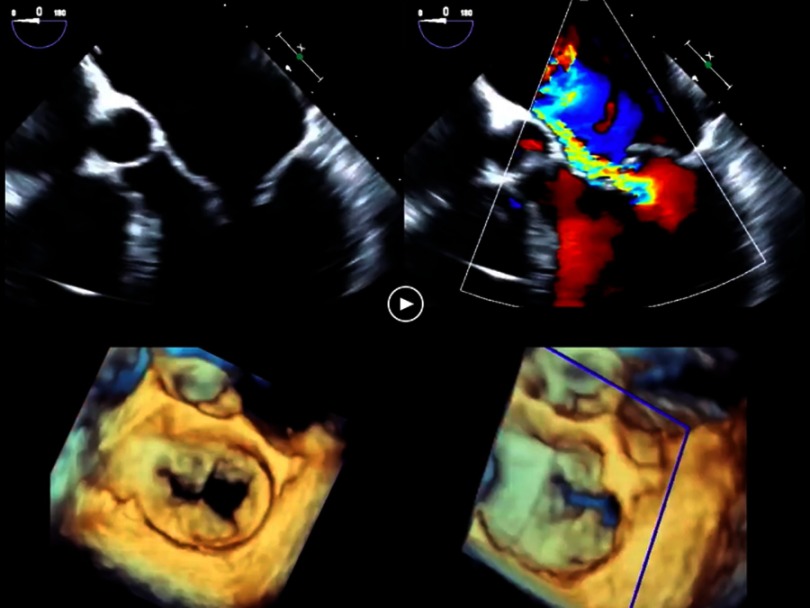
The TEE demonstrates fibroelastic deficiency with prolapse and flail of the P2 scallop shown in 2D imaging (upper left), with color Doppler of the eccentric mitral regurgitation (upper right) and 3D TEE imaging en face from left atrium (lower right) and tilted laterally (lower left). (“Movie files are available at https://globalcardiologyscienceandpractice.com ”)
